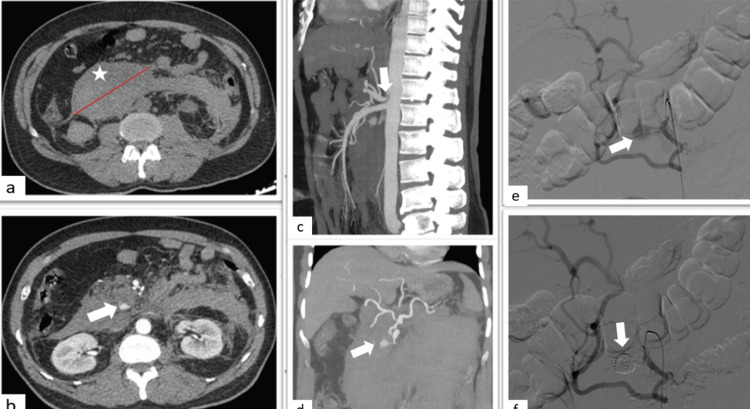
Abdominal CT and diagnostic/therapeutic mesenteric angiography in a case of spontaneous mesenteric hematoma secondary to a pancreaticoduodenal artery aneurysm with median arcuate ligament compression. Multiphase abdominal CT angiography shows a large spontaneously hyperdense mesenteric hematoma measuring approximately 24 cm (a) and an aneurysm arising from a branch of the pancreaticoduodenal arcade originating from the superior mesenteric artery (arrows in b and d). Sagittal arterial-phase reconstruction demonstrates moderate compression of the celiac trunk by the median arcuate ligament (c). Selective mesenteric angiography confirms the aneurysm (arrow in e), which was successfully treated by coil embolization (arrow in f).
